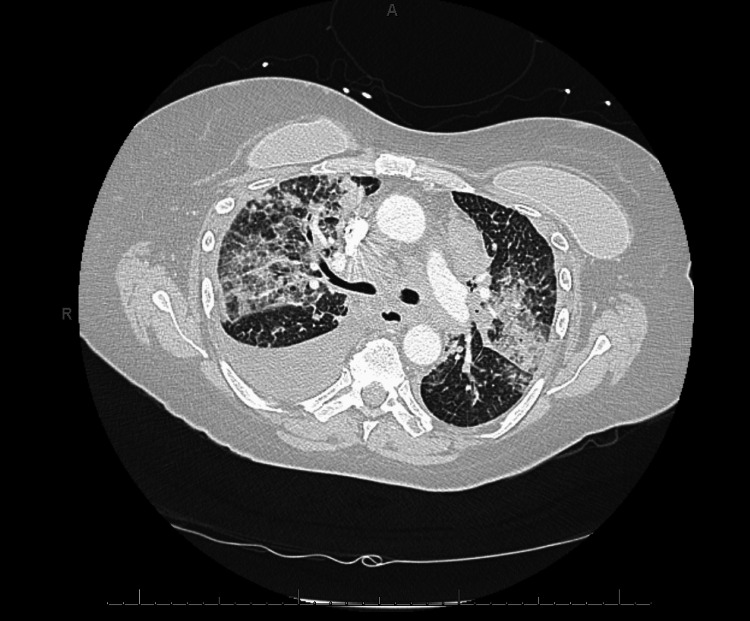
CT chest at the outside hospital CT: computed tomography
